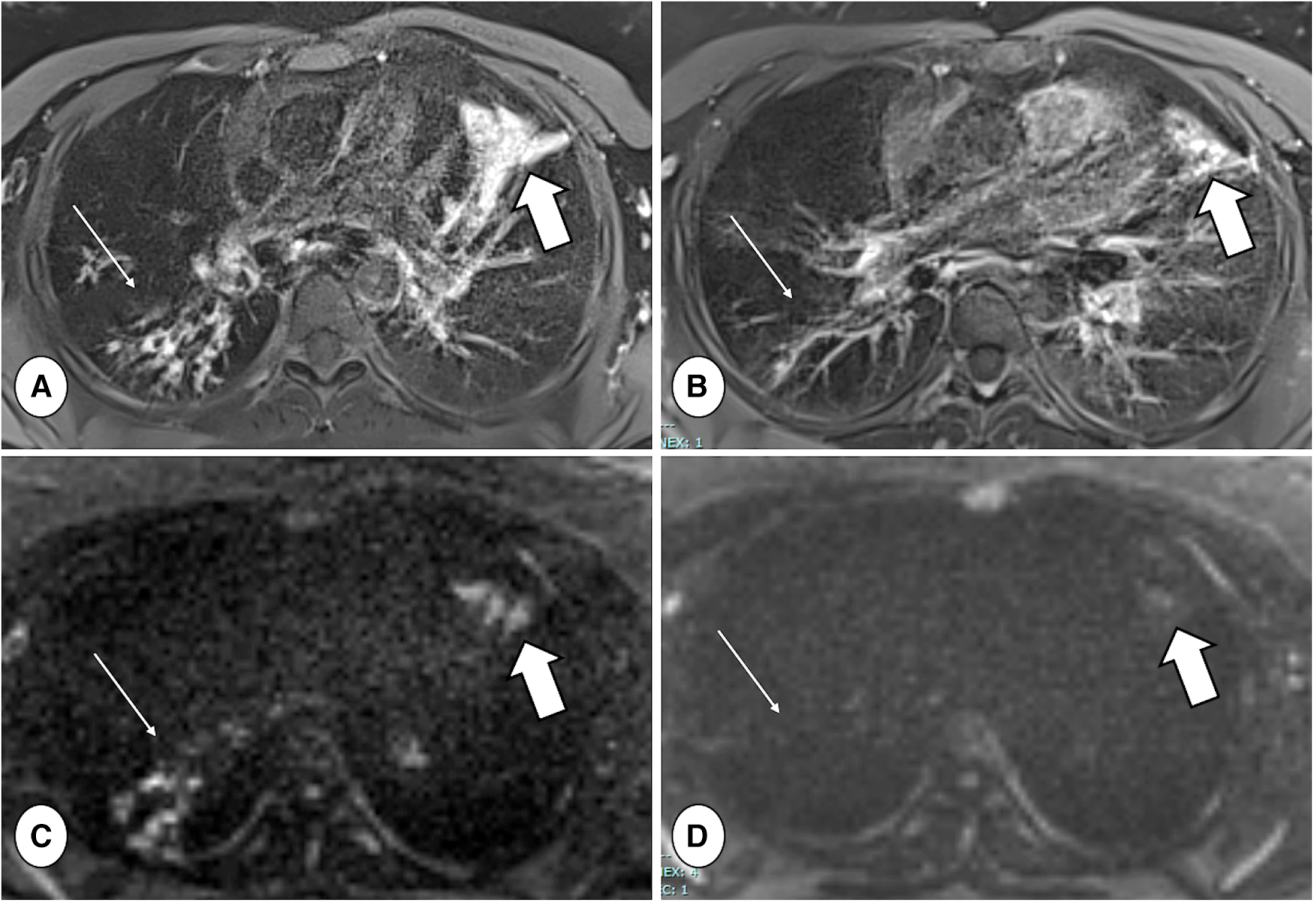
Axial proton density-weighted PROPELLER free-breathing scan (A,B) and diffusion-weighted imaging (DWI) (b = 800 mm/s^2^) acquisition (C,D), both performed with a 1.5 T MRI system (MAGNETOM avanto, siemens healthineers, enlargen, Germany), in a patient with CF during respiratory tract exacerbation at baseline (A,C) and after treatment (B,D). Note the reduction in mucus plugs and DWI signal in the right lower lobe between baseline and follow-up (thin arrows), as well as the reduced consolidation and DWI signal in the lingula (thick arrows).
